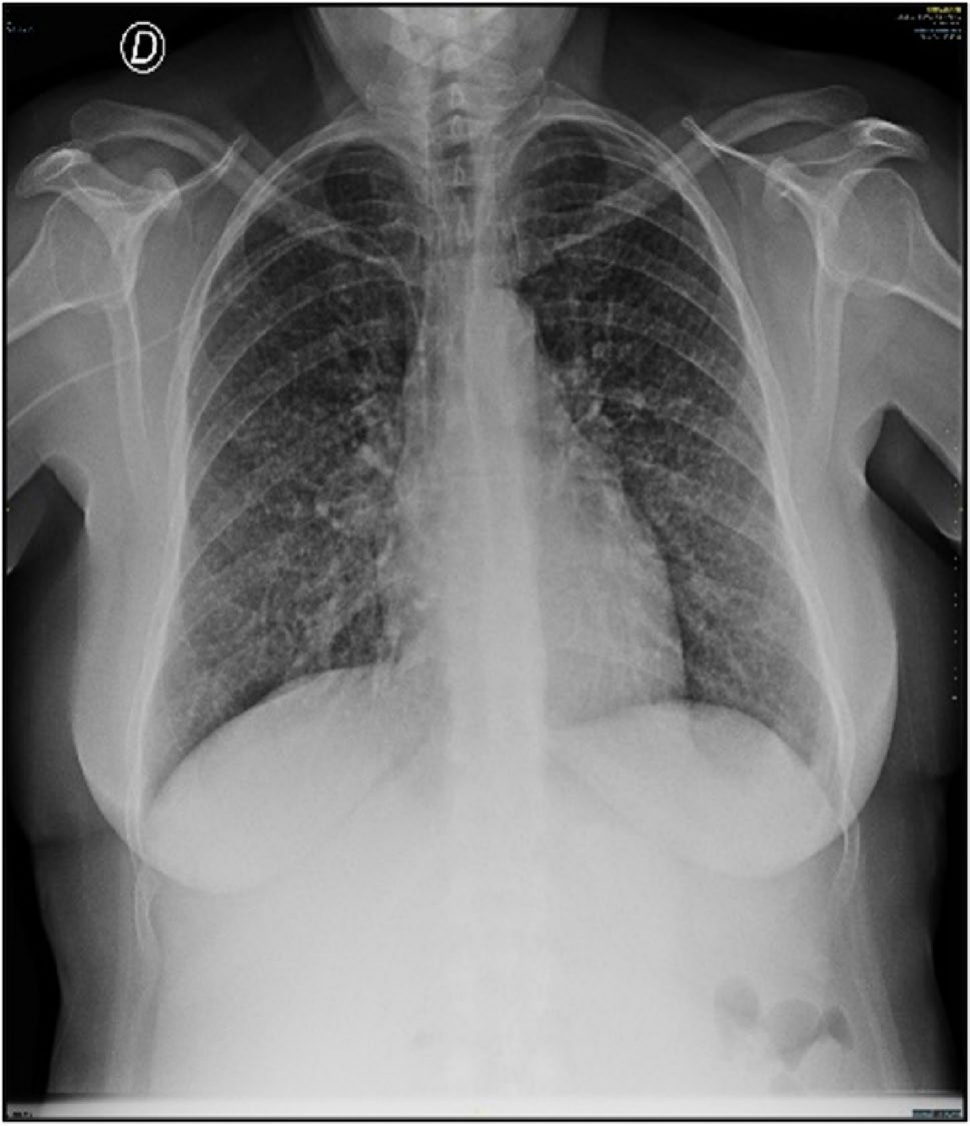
Bilateral ground-glass opacities, predominantly at the lung bases, were evident on the chest X-ray
